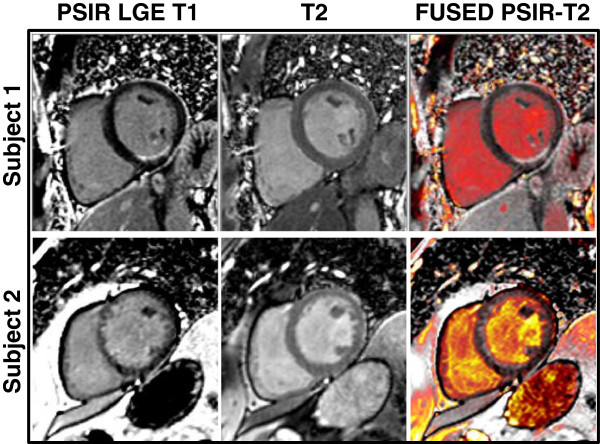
Fusion of MCODE T1 and T2 data: DICOM images from MCODE sequences can be loaded into a free open source software, OsiriX Imaging Software. From the MCODE sequence, the T2-weighted image is fused with the PSIR image with the Image Fusion function. No manual registration of the images is necessary since they were acquired during the same breath hold. When fused, the grey-scale T2-weighted image is then converted to the PET color look up table and overlays the grey-scale PSIR image. The image is then windowed and leveled to display areas with low T2 signal intensity (myocardium) to appear as dark red, and areas with high T2 signal intensity (blood pool) to appear as bright yellow.
